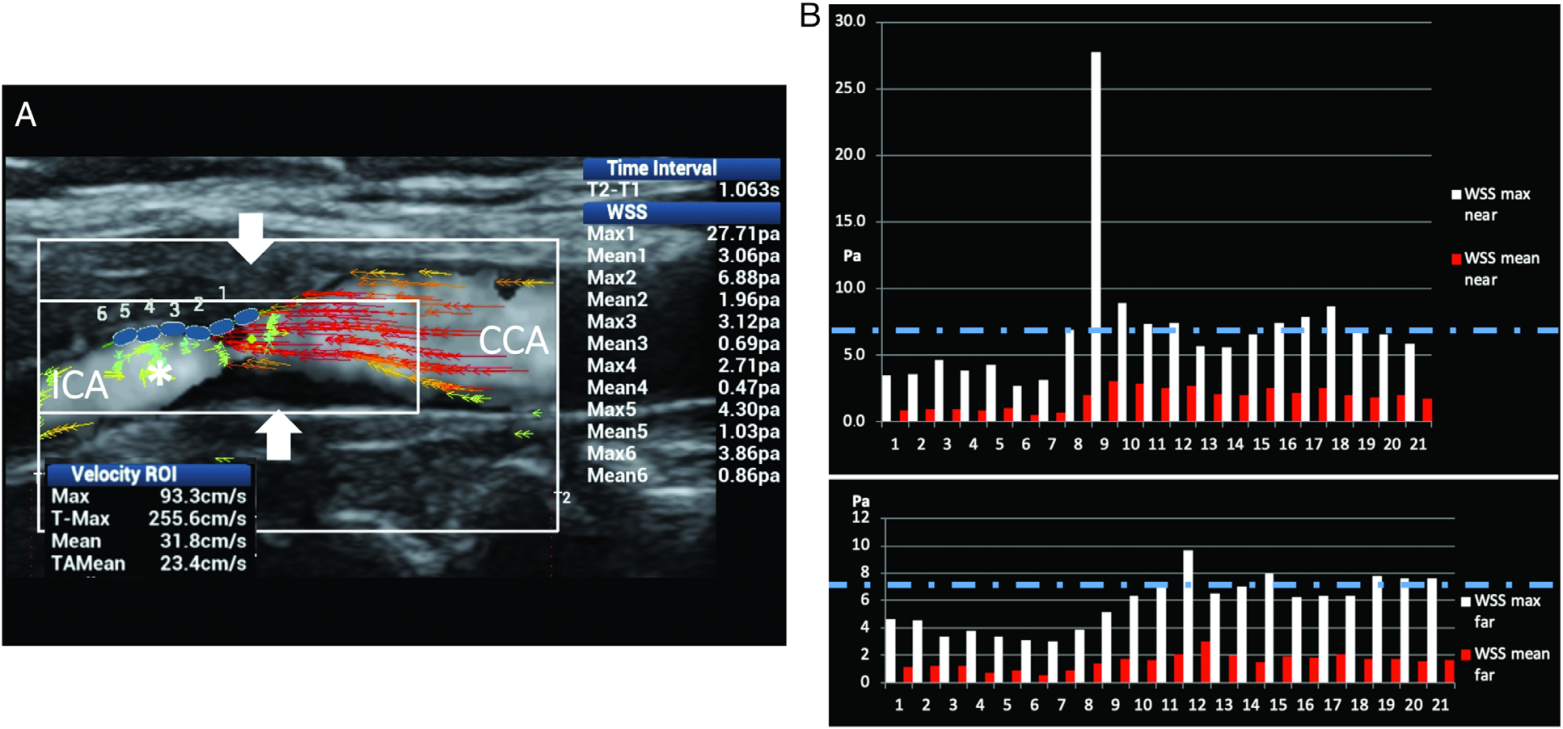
Substantial carotid stenosis. A, Ultrasound vector flow imaging shows very high‐velocity red vectors (maximum velocity along time in the region of interest, 255 cm/s) at the level of the stenosis (arrows) and substantial turbulence distally to the stenosis (asterisk). B, The graphs obtained by measuring WSS point by point along the near and far vessel walls show very high WSS maximum values of 27.7 and 9.69 Pa, respectively (dashed line corresponds to the maximum normal value) at the level of the stenosis. CCA indicates common carotid artery; and ICA, internal carotid artery.
